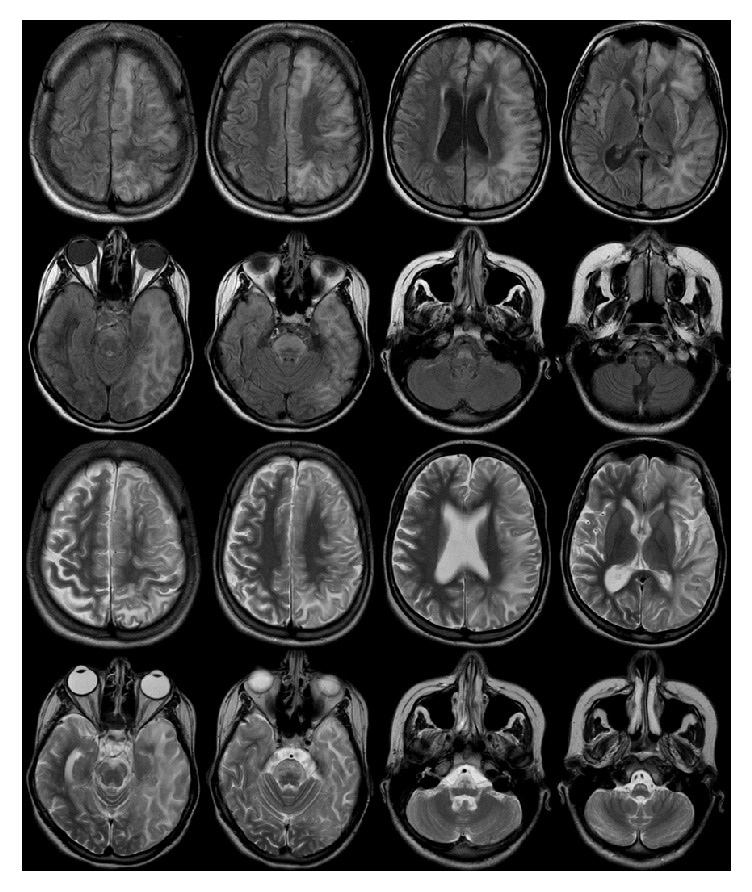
MRI of the brain showing evidence of diffuse asymmetrical, left more than right, cortical laminar necrosis secondary to refractory status epilepticus with anoxic brain damage. The images are FLAIR (upper two rows) and T2-weighted images (lower two rows). The changes are high signal intensity involving both supratentorial structures and brain stem.
